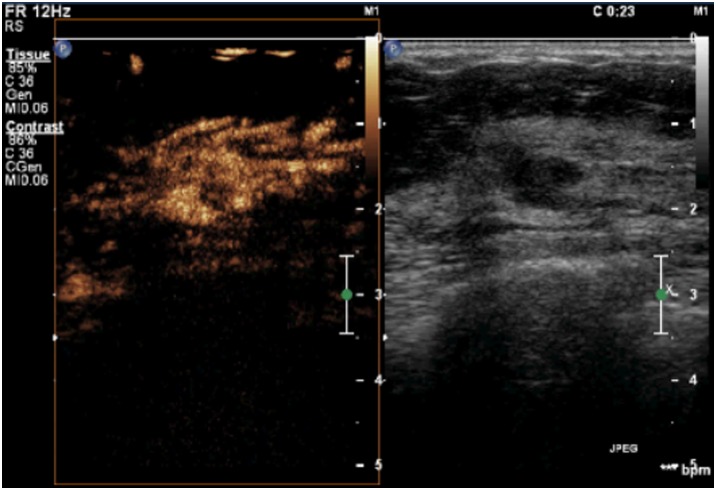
A lesion with a score of 5 by breast contrast-enhanced ultrasound. The lesion is heterogeneously enhanced, with a larger region (compared with that of a 2D image), earlier enhancement, and with/without perfusion defect, particularly with a typical crab claw-like enhancement and an unclear borderline.
